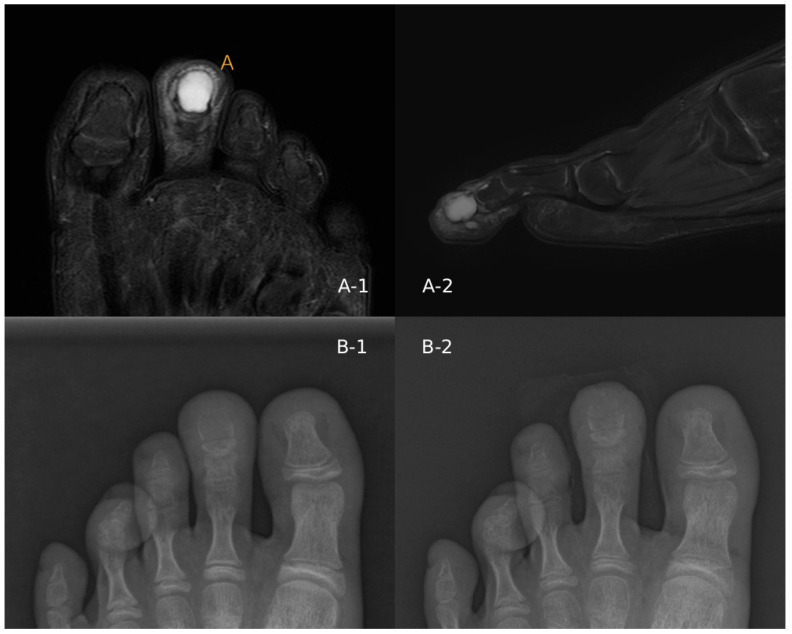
Preoperative imaging findings. (A-1) Axial FS-T2 MR: expansile lesion confined to the distal phalanx. (A-2) Sagittal FS-T2 MR: lesion extent. (B-1) Plain radiograph: osteolytic lesion with cortical expansion, no cortical destruction. (B-2) Postoperative radiograph after curettage and bone grafting.
